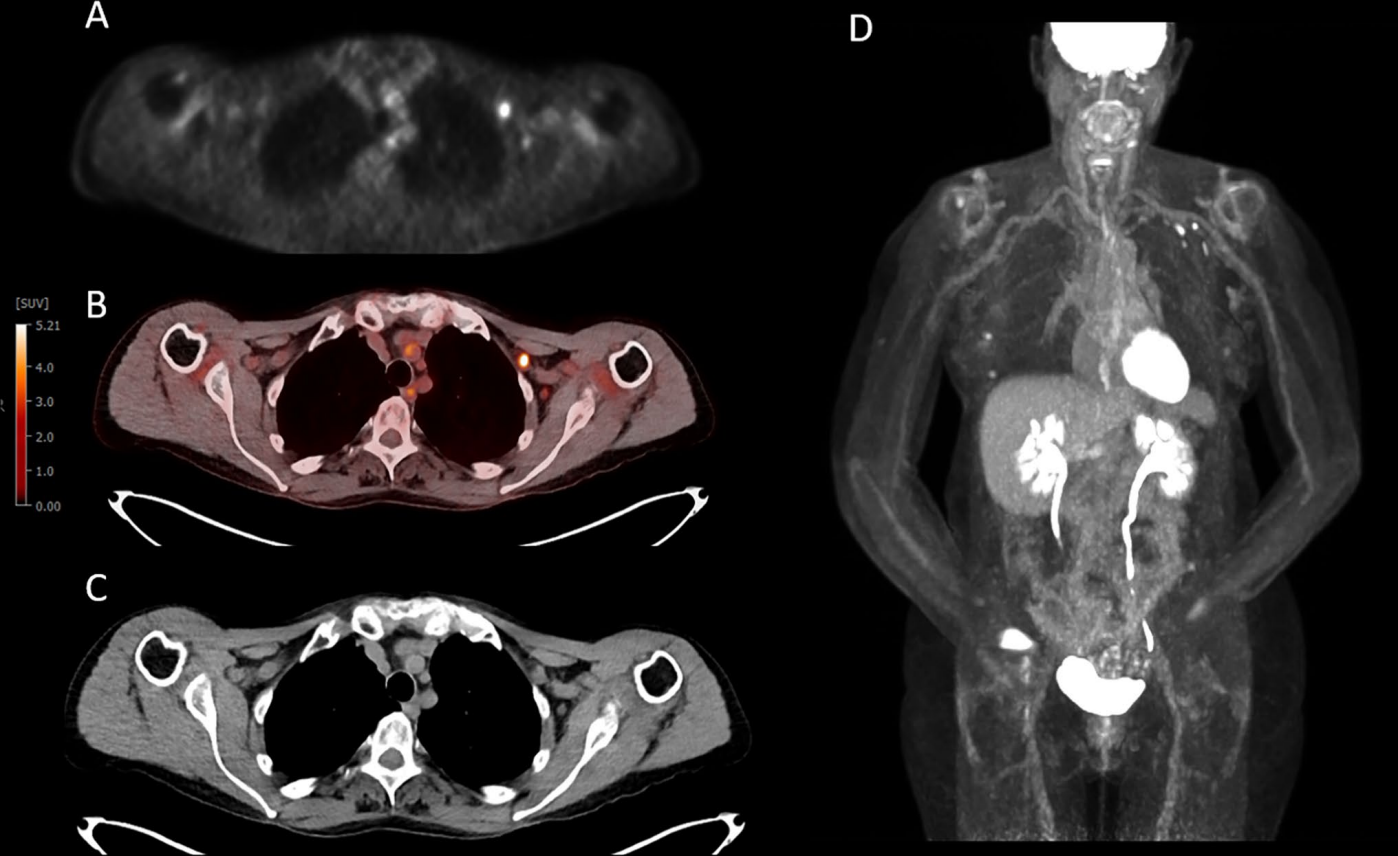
Selected images from serial FDG PET/CT examinations for a patient who received 2 doses of Moderna vaccination, with the second dose approximately 1 month before initial imaging. Trans-axial FDG PET (A) fused FDG PET/CT (B) transaxial non-contrast CT image (C) and FDG PET MIP (D) show two non-enlarged but hypermetabolic left axillary lymph nodes, ipsilateral to the COVID-19 vaccination sites in the left arm. ΔSUVmax was 6.7 and 6.8, in the anterior and posterior nodes, respectively
